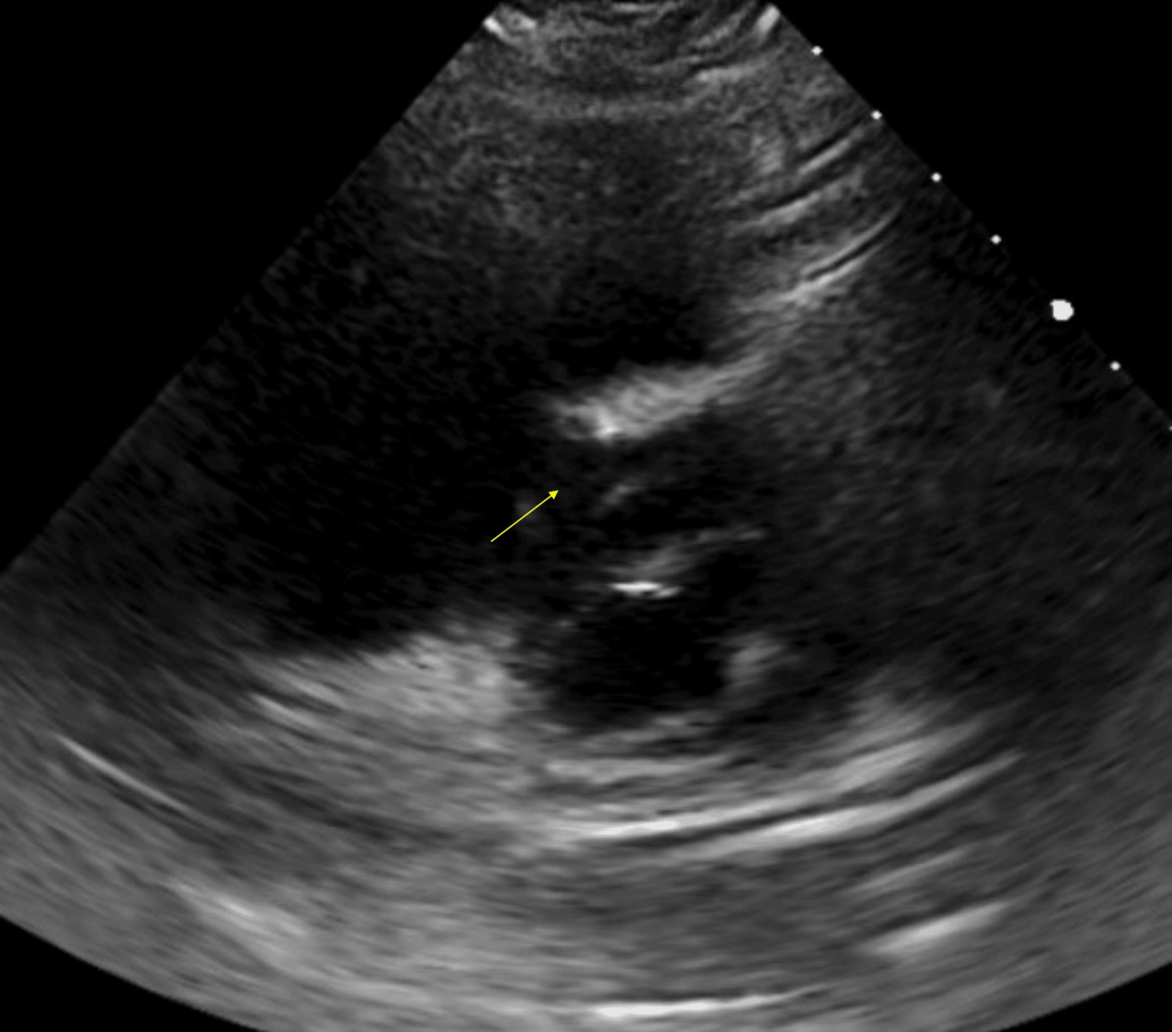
A transthoracic echocardiogram on hospital day four in parasternal short view demonstrated interventricular septal flattening (yellow arrow) during systole and right ventricle dilation consistent with acute pulmonary embolus.
